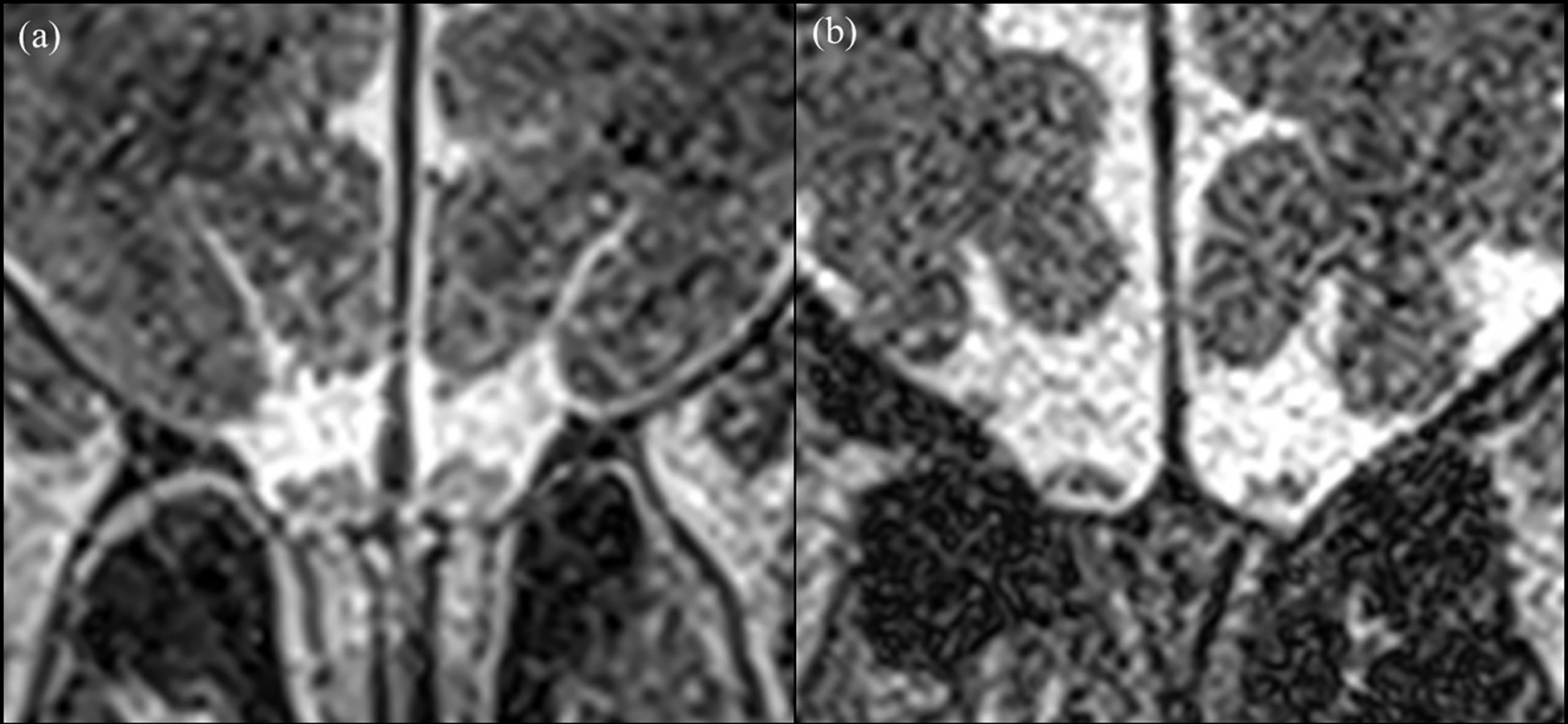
Representative cases. (a) Images obtained from a 64-year-old man with idiopathic Parkinson’s disease (iPD), with modified Hoehn and Yahr scale stage (HY) 1. On the coronal T2-weighted image, each left and right olfactory bulb was observed, and the average of olfactory bulb height (OBH) was 1.72 mm. (b) On the image of an 84-year-old female iPD patient with HY3, the measured OBH was 1.16 mm.
